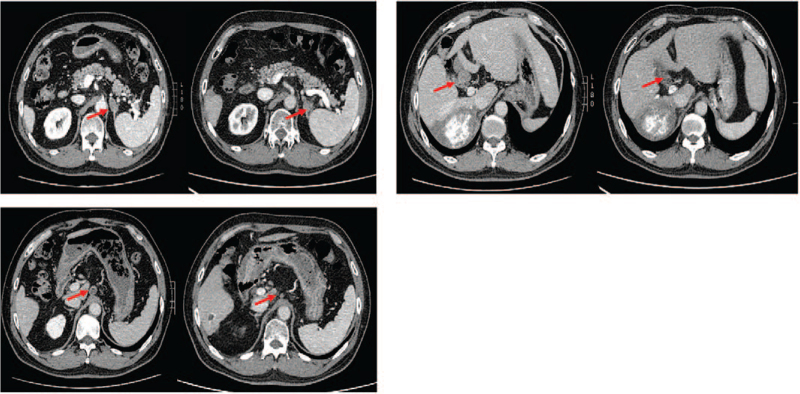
After the second IGRT, enhanced CT showed that the left adrenal gland metastasis, the lymph nodes of the hepatic hilar and retroperitoneal became obviously smaller. CT = computed tomography, IGRT = image-guided radiotherapy.
